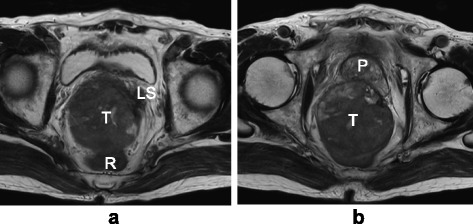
Magnetic resonance imaging T2-weighted images: Tumor invasion of the a left seminal vesicle and b prostate was suspected. *LS* left seminal vesicle, *P* prostate, *R* rectum, *T* tumor
